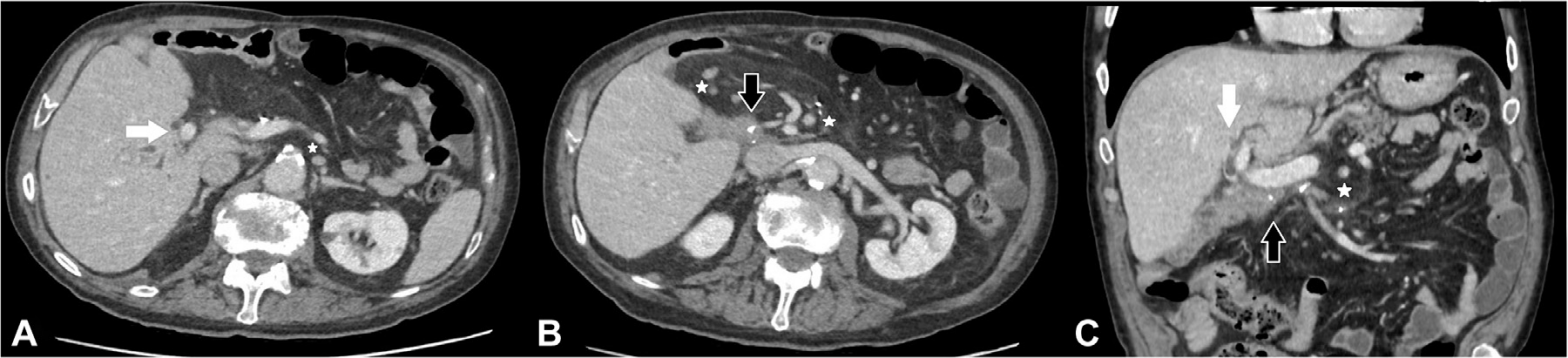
(A-C) Patient with a history of cholangiocarcinoma treated by pylorus-preserving pancreaticoduodenectomy with biliodigestive anastomosis. After several recurrent episodes of cholangitis, CT shows intrahepatic cholestasis with cholangitis of the distal courses of the right and left hepatic ducts (white arrows). Inflammatory changes of the BDA without evidence of insufficiency (black arrows). There is also an increased number of paraaortic, interaortocaval and retrocaval lymph nodes measuring almost 12 mm (white star).
